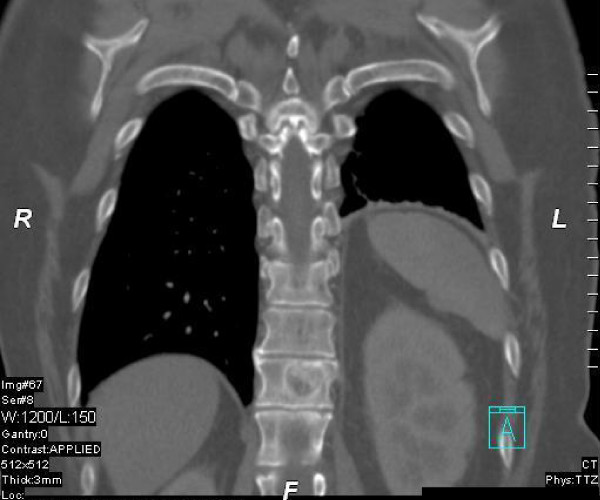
Coronal CT scan of the vertebral metastasis.
